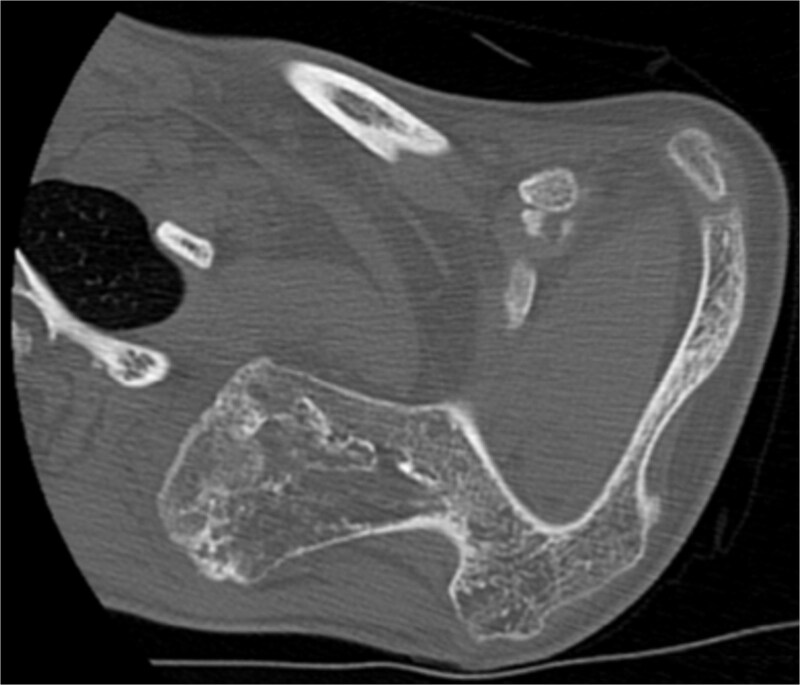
CT of the 14-year-old patient showing the largest lesion arising ventrally from the superior angle of left scapula in axial plane.
